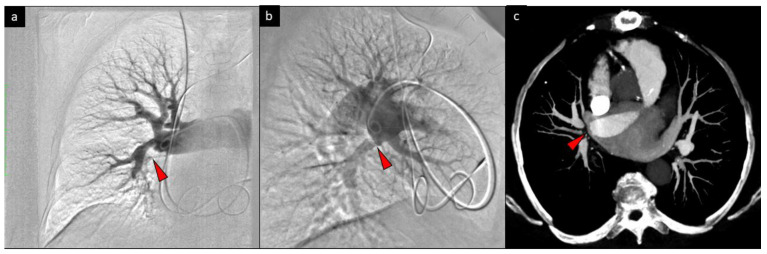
Angiography of the right pulmonary artery with a proximal thromboembolic lesion (marked by the red arrow) in the anteroposterior view (a), lateral view (b), and computed tomography (c).
